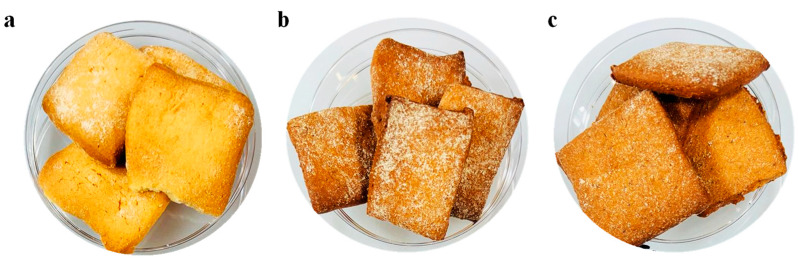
Cookies samples analysed in the sensory panel: (a) CTRL-C (control); (b) HAD-Cookies (hot air drying); (c) US-HAD-Cookies (ultrasound-assisted hot air drying).
